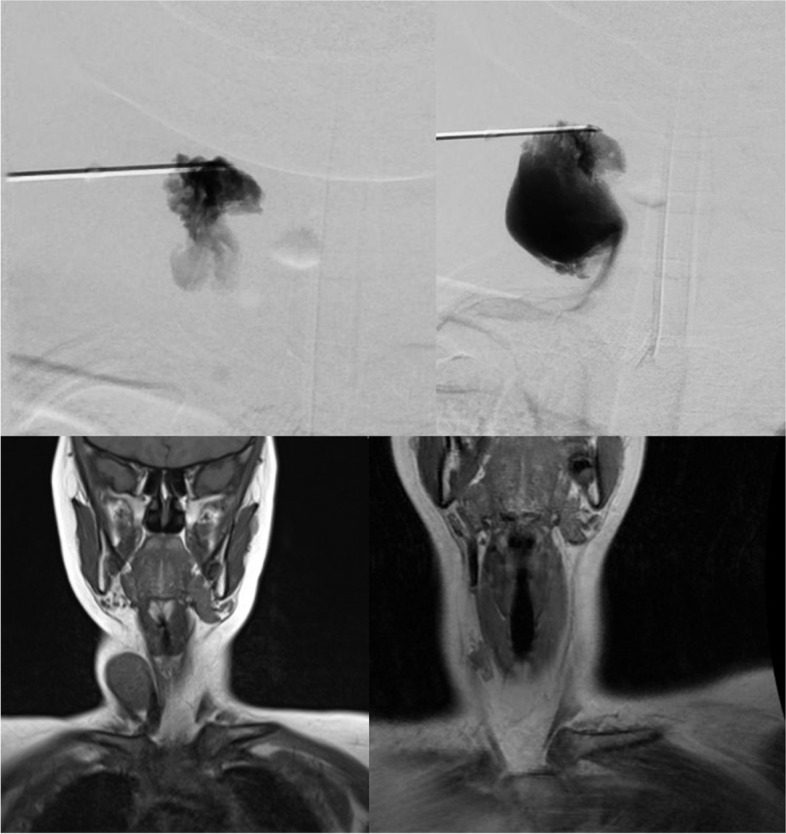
Patient with a VM in the neck area. Above: Phlebographic findings before the intervention. Bottom: MRI findings before the intervention (left) and after (right)
